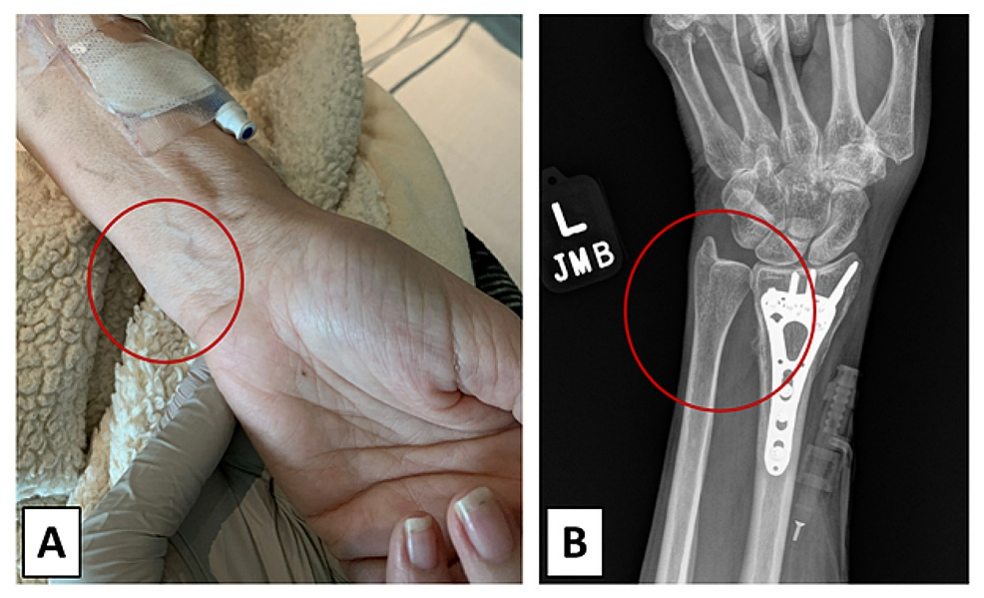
Investigation of the left wrist for infection on hospital day 8. (A) Gross observation of left wrist and (B) X-ray of patient's left wrist.
